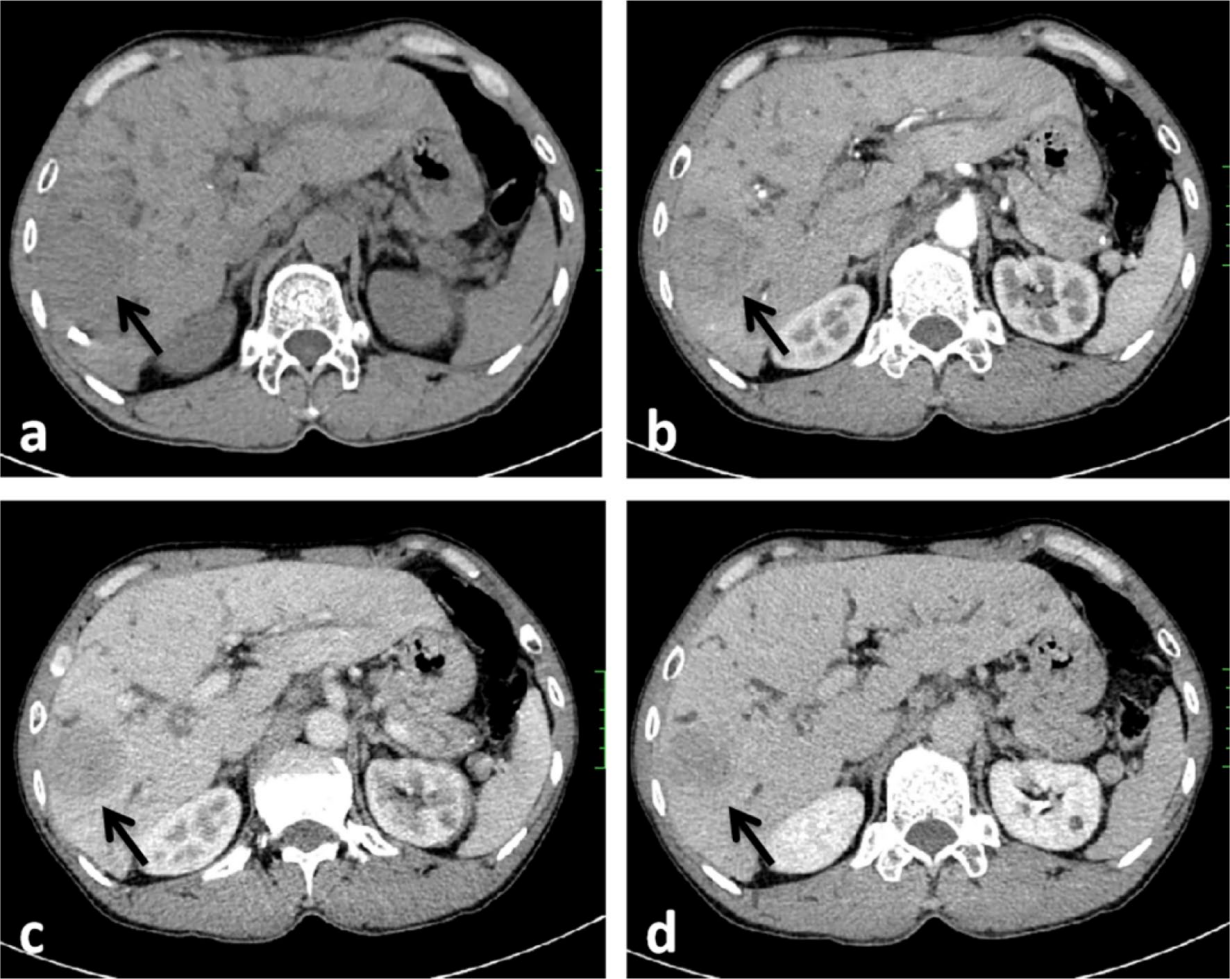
CT images in a 54-year-old HBV-negetive man with ICC confirmed by surgical resection, positive for schistosoma hepatica, AFP 6.56 ng/ml, CA19-9 < 2.00 U/ml, and its enhancement pattern was categorised as type II. Precontrast image (a) showed a solitary tumor (arrow) in segment VI of the liver; arterial phase (b), portal phase (c) and delayed phase (d) showed progressive hypoenhancement; portal phase (c) showed dilated intrahepatic bile ducts.
